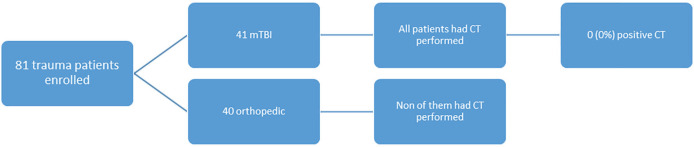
Forty orthopedics’ patients and 41 mild traumatic brain injury (mTBI) patients were enrolled into study, none one these patients had positive brain brain computed tomography (CT) lesion
